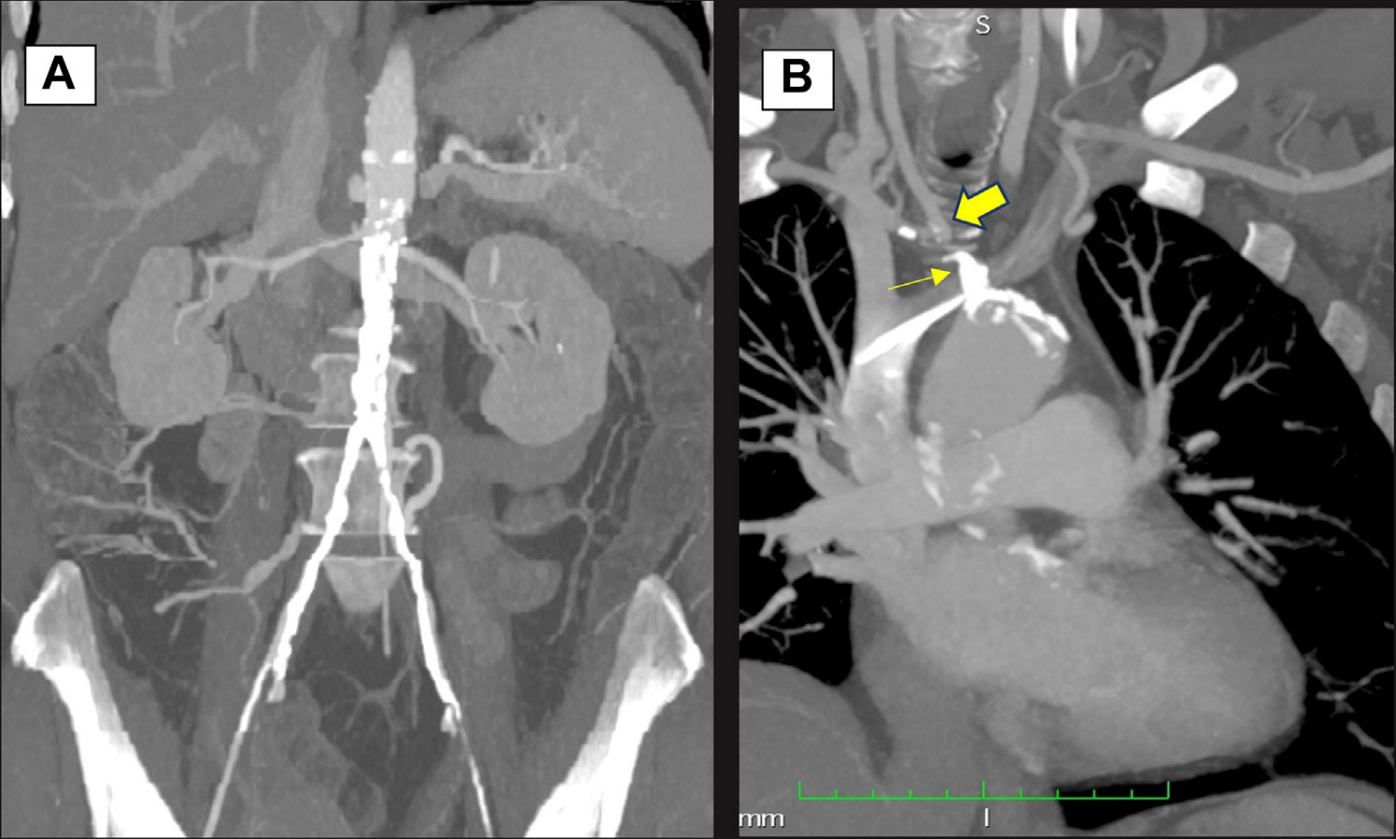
Coronal computed tomography angiographic views showing extensive calcified occlusion of the juxtarenal aorta (A) and brachiocephalic artery (BCA; B, *narrow arrow*), with a patent carotid artery–subclavian artery bifurcation (*large arrow*).
